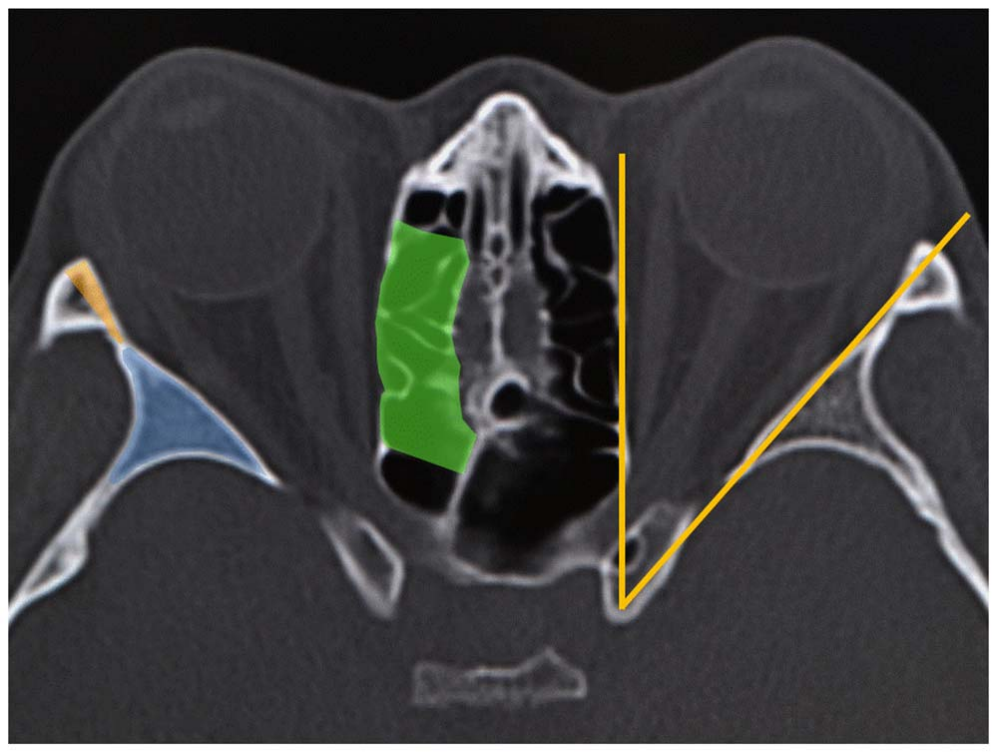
Axial computed tomographic image of the orbit. In the lateral orbital wall decompression, the greater wing of the sphenoid (the blue area), and “the medial eaves” of the lateral orbital rim (the orange area) were removed. In the medial orbital wall decompression, the medial orbital wall with its periosteum and the septa of the ethmoid air cells (the green area) were removed. The lateral walls are oriented to an angle of 45 degrees lateral to the sagittal plane, whereas the medial walls of each orbit are oriented in the sagittal plane (the yellow solid lines).
